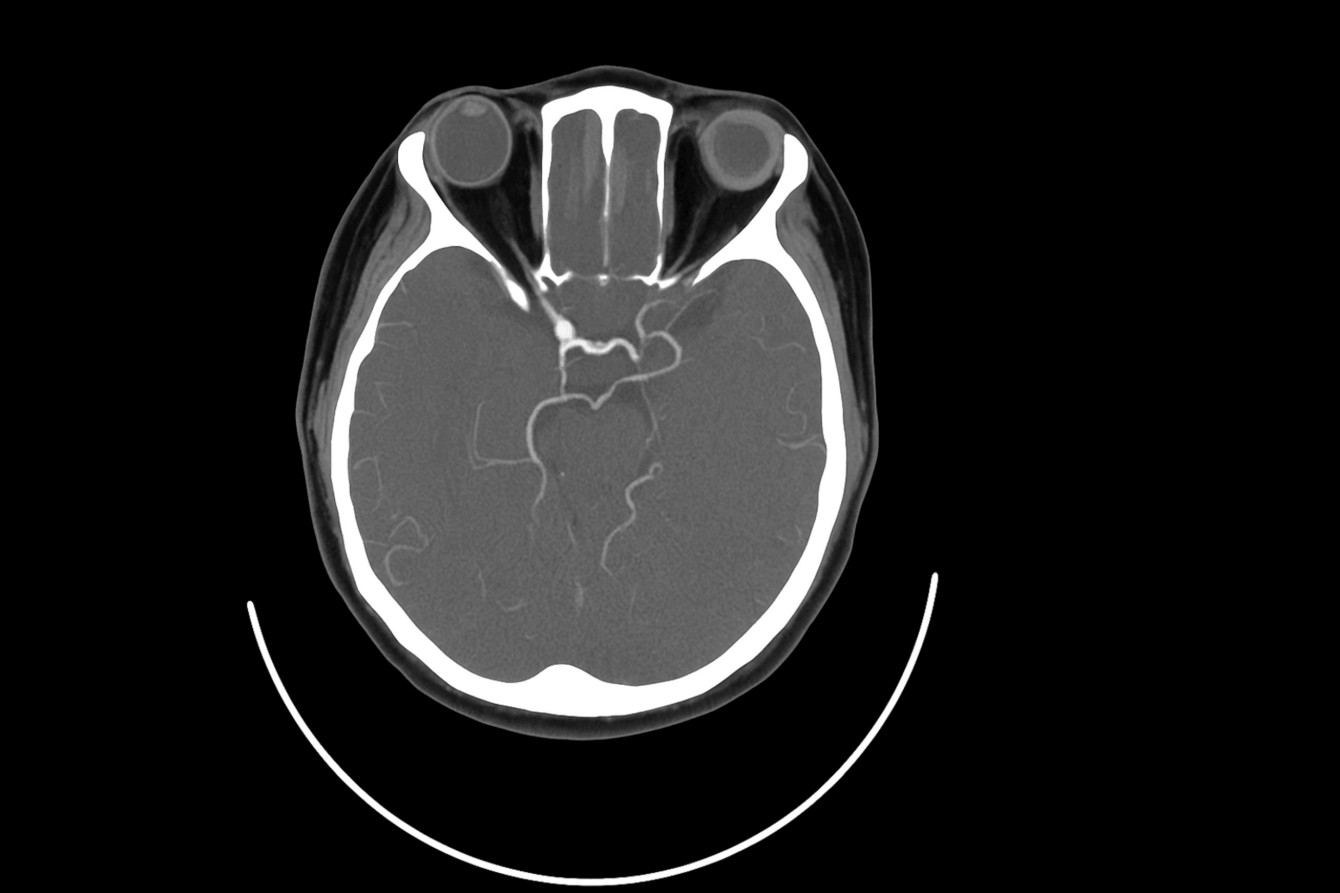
CT angiogram (axial view): normal circle of Willis. No carotid dissection.
